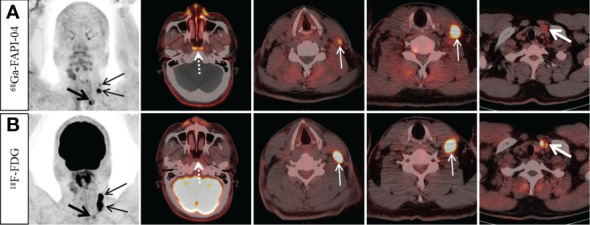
A 49-year-old man with nonkeratinizing differentiated carcinoma. ^68^Ga-FAPI-04 PET/CT showed intensive ^68^Ga-FAPI-04 uptake in the posterior nasopharyngeal wall (A, dotted arrow, SUVmax 3.8), ^18^F-FDG PET/CT showed no abnormal ^18^F-FDG uptake in the primary tumor (B, dotted arrow). Moreover, ^68^Ga-FAPI-04 PET/CT reveals higher tracer uptake than ^18^F-FDG PET/CT in the left supraclavicular lymph node (A, thick arrow, SUVmax 9.4 vs. B, thick arrow, SUVmax 3.8), but the tracer uptake of left cervical (level III) lymph nodes was lower than that of ^18^F-FDG PET/CT (A, thin arrow, SUVmax, 4.5–11.0 vs. B, thin arrow, SUVmax, 17.7–19.4).
